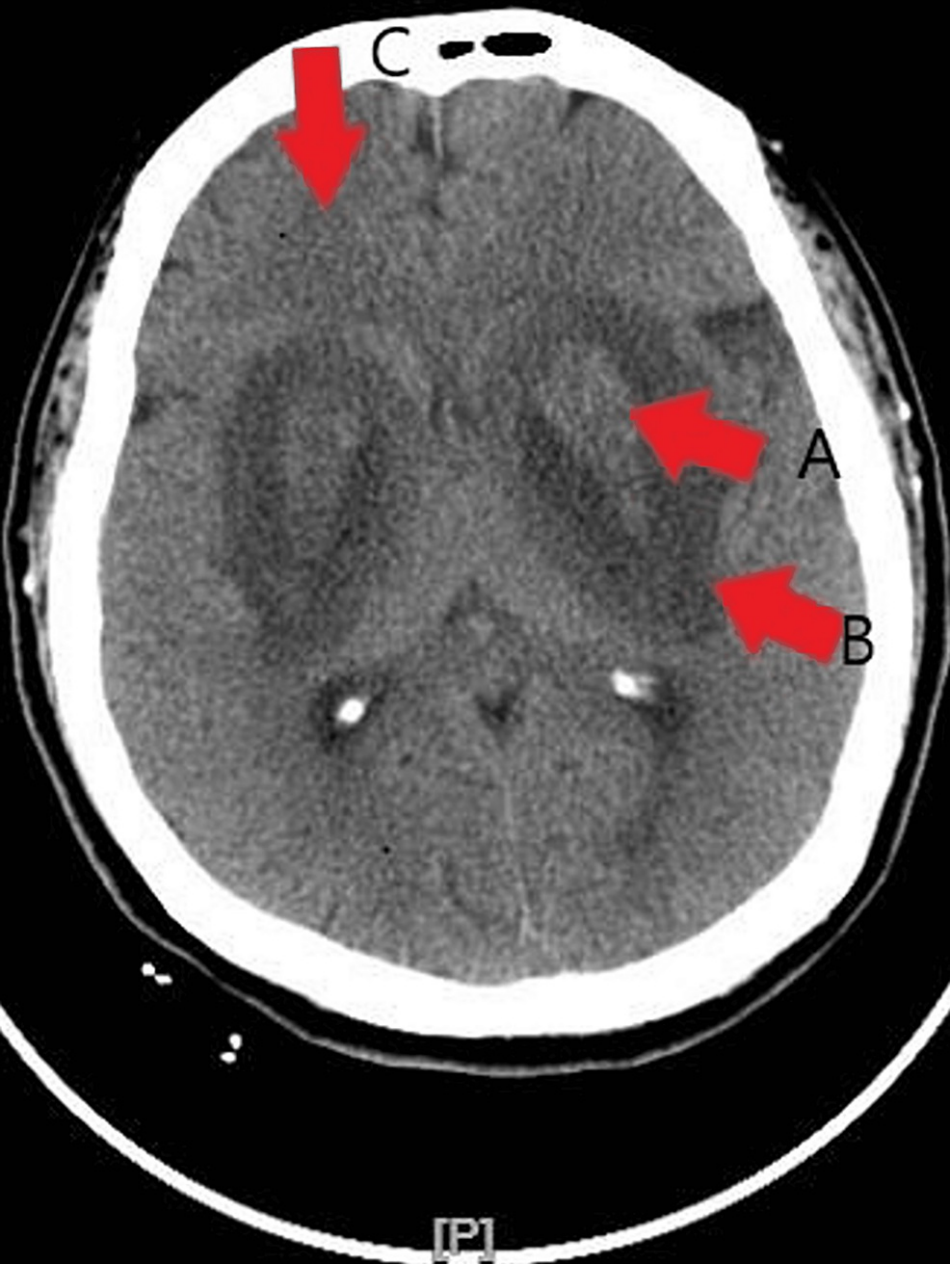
Initial CT head without contrast revealing basal ganglia infarct and possible lentiform nuclei hemorrhaging. Multiple contiguous axial images were obtained from the skull base to the vertex without the administration of intravenous contrast. Sagittal and coronal 2D reformations were performed. There are areas of lucency in the temporal lobes bilaterally involving the lentiform nuclei (Arrow B). There are patchy hyper-densities of the lentiform nuclei bilaterally suggesting hemorrhage (Arrow A). There is marked lucency surrounding the basal ganglia on both temporal lobes (Arrow B), left caudate nucleus (Arrow B), adjacent bilateral frontal (Arrow C) and parietal lobes (Arrow C) suggesting ischemia or anoxic changes. There is no extra-axial collection or midline shift. There is a mass effect on the lateral ventricles especially the frontal horns and temporal horns without hydrocephalus.
